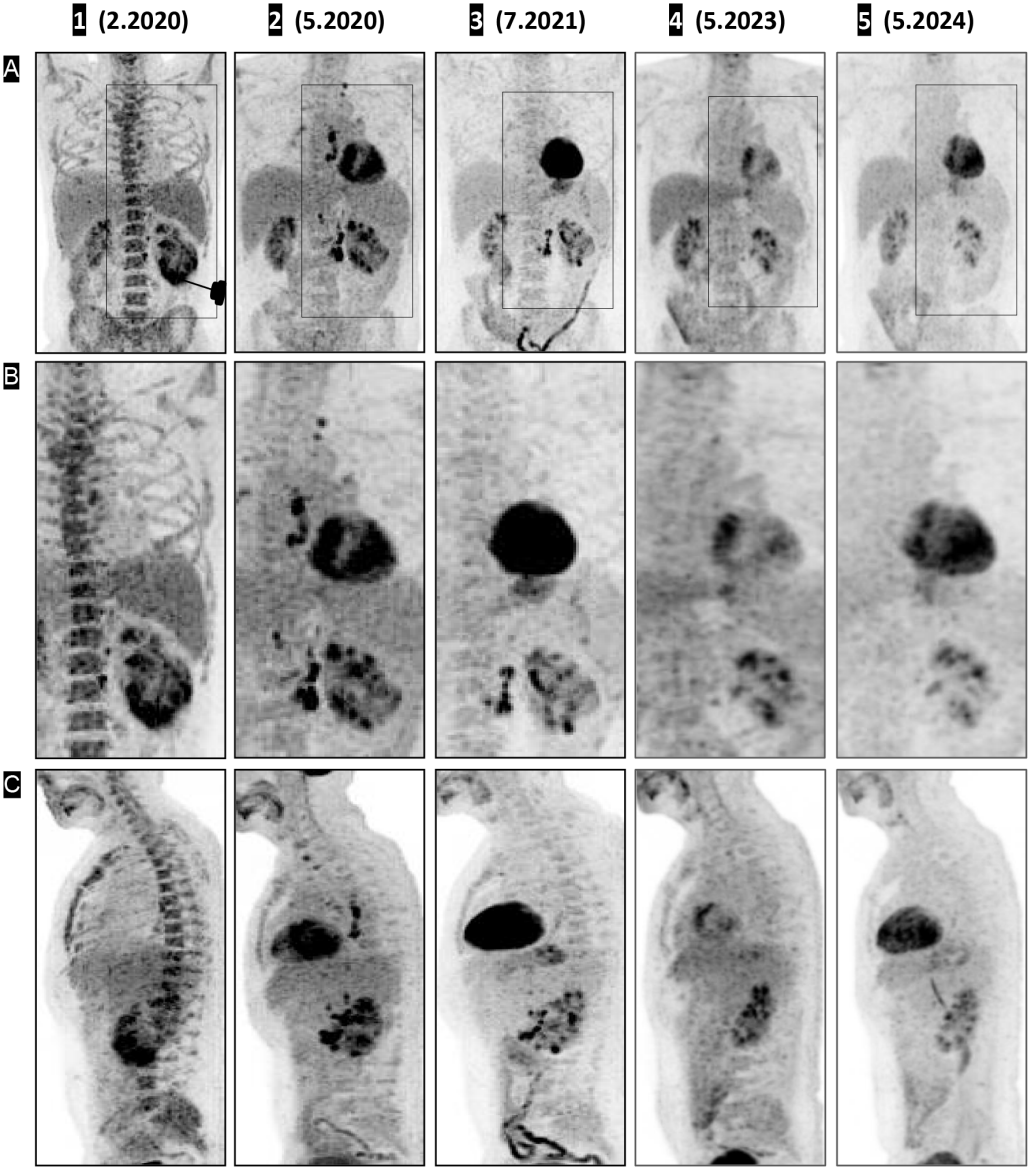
CT, PET, and fused PET-CT images demonstrating shrinkage of the renal mass (coronal view) and urine cast of necrotic tumor tissue. The treatment response timeline of the left renal primary lesion is shown in coronal view panels as contrast-enhanced CT (A 1–5), fused PET (B 1–5), unfused PET CT (C 1–5), and 3D volume-rendered images using angio (D 1–5) and soft-tissue (E 1–5) presets with digital subtraction of background anatomy. Contrast CT on (A 1), fused PET CT on (B 1), and unfused PET on (C 1) shows a large left renal mass with intense pathological uptake, followed by a decrease in size on (A 2, 3) and a small residual scar on (A 4). On (A 5), there is interval development of a possible focus of dystrophic calcification. (B 2–C 2, B 3–C 3) Significant decrease in uptake, with complete resolution of pathological uptake on (B 5–C 5). The 3D volume-rendered images in the angio preset (D 1) and soft-tissue preset (E 1) show PET-correlated intense uptake in the lower pole, appearing as a thickened lower pole cortex. (D 2–E 2) Decreased uptake with a central deficit corresponding to necrotic debris; (D 3–E 3, D 4–E 4, D 5–E 5) Restored physiologic uptake.
